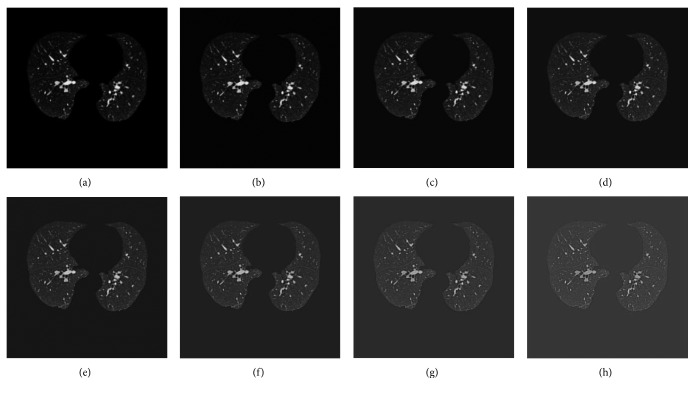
The result of different differential order: (a) original CT image, (b) 0.1 order, (c) 0.2 order, (d) 0.3 order, (e) 0.4 order, (f) 0.5 order, (g) 0.6 order, (h) 0.7 order.
